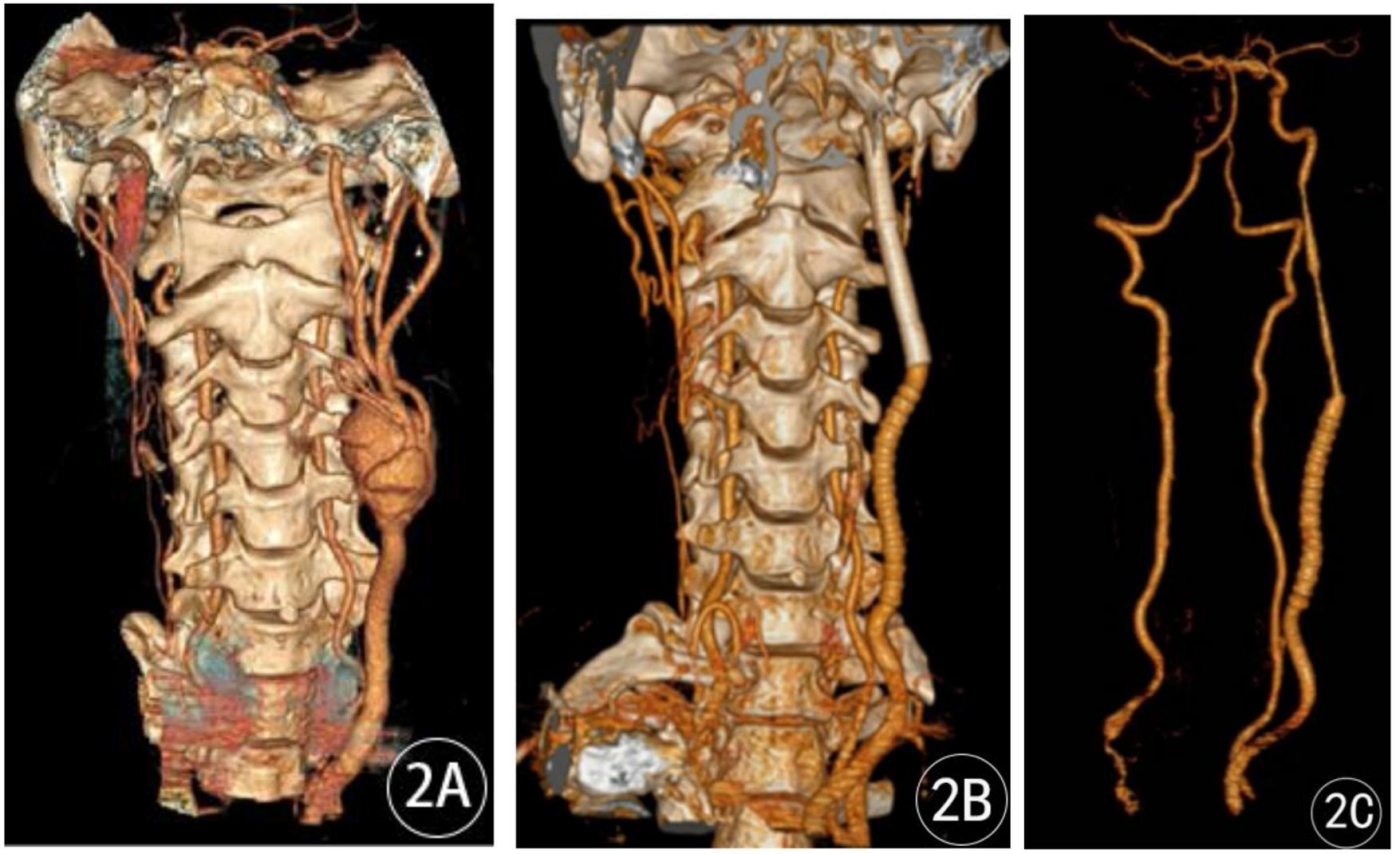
Three-dimensional reconstructions of CTA of the neck: (A) before the operation and (B,C) 10 days after the operation.
